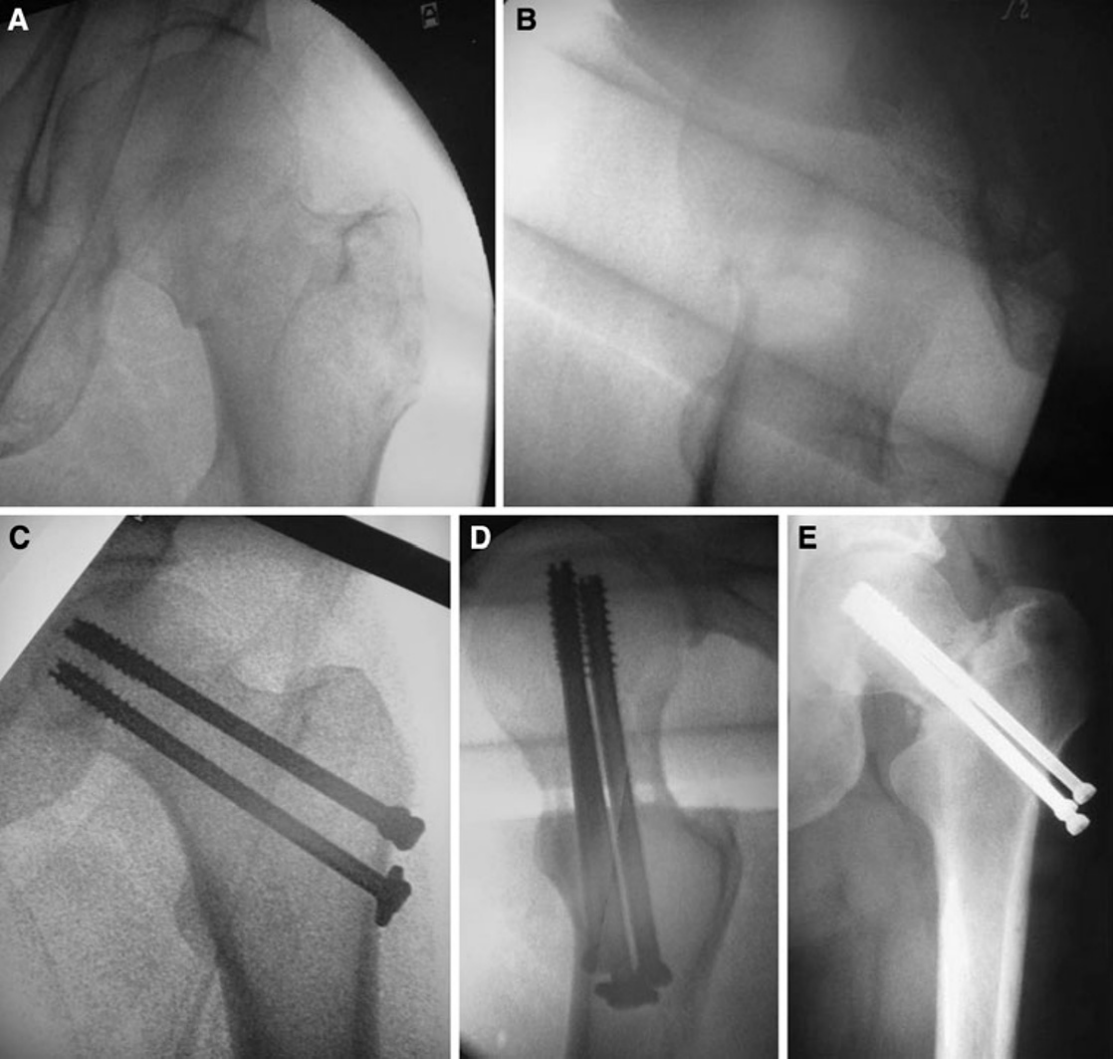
Intraoperative a anteroposterior and b lateral fluoroscopy views show a non-displaced Garden II femoral neck fracture in a 64-year-old woman. Intraoperative c anteroposterior and d lateral fluoroscopy views after internal fixation with cannulated hip screws. e At 8 months, anteroposterior radiograph of the hip of the patient shown in Fig. 1 shows resorption of the femoral neck and lateral migration of the hip screws, suggesting non-union of the femoral neck fracture. Revision to total hip arthroplasty was done
